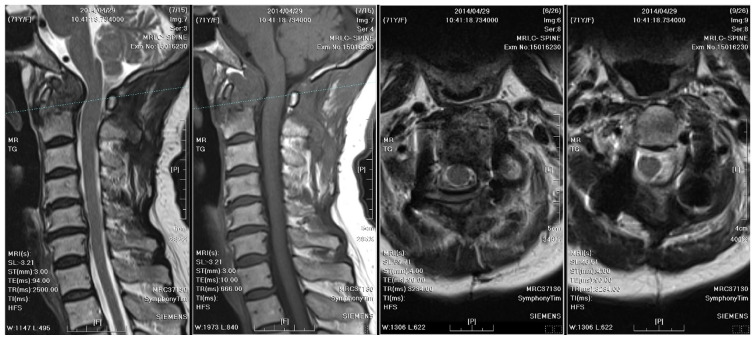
A follow-up cervical spine MRI taken 10 months after posterior surgery revealed resolution of the atlantoaxial subluxation; however, fibro-osseous hypertrophy at the atlanto-odontoid joint led to stenosis of the spinal canal.
